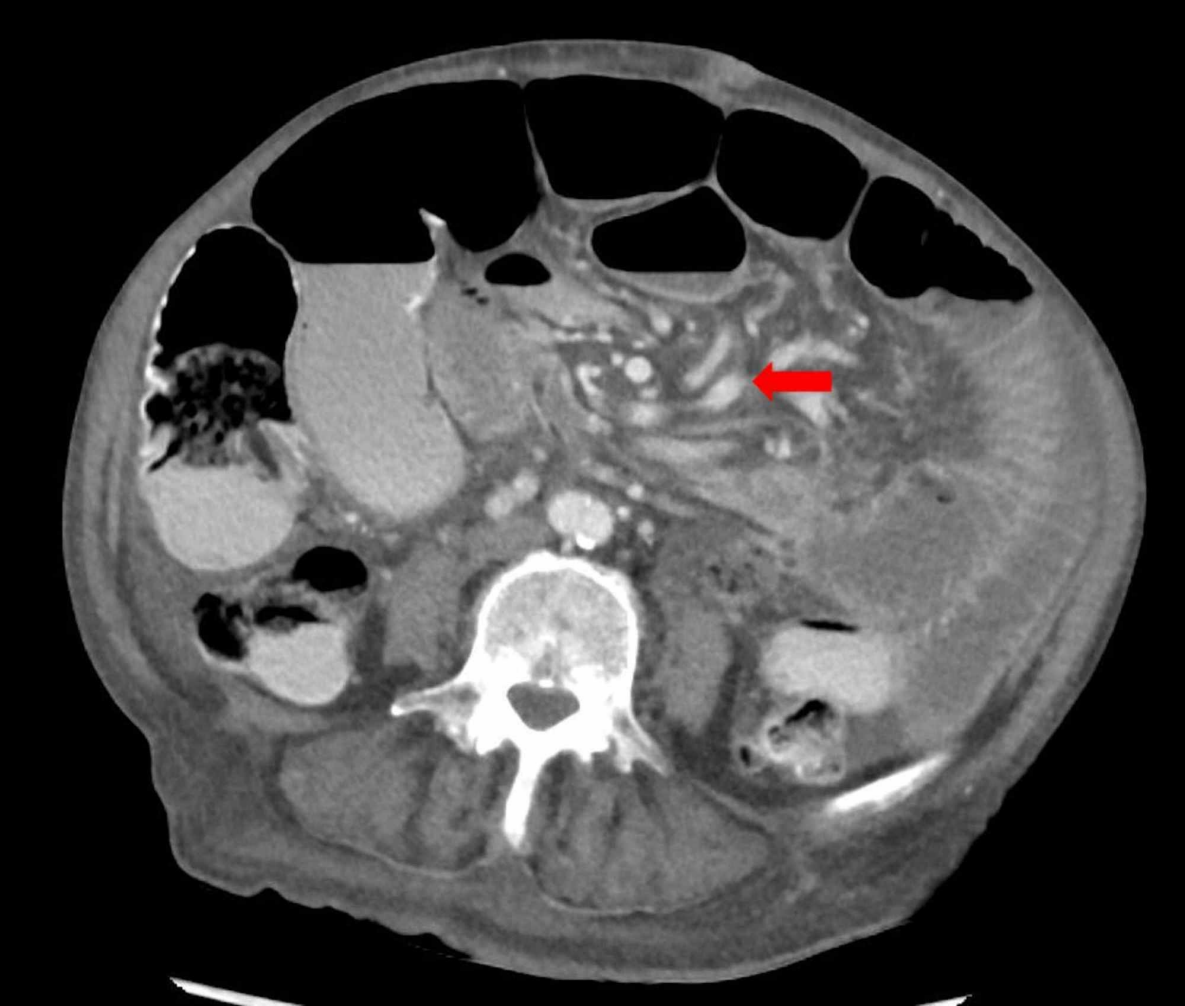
Post-operative axial slice of abdominal computed tomography (CT) in portal phase demonstrating recurrence of “whirlpool sign” of mesenteric vessels, suggestive of small bowel volvulus (arrow) with dilated loops of small bowel
